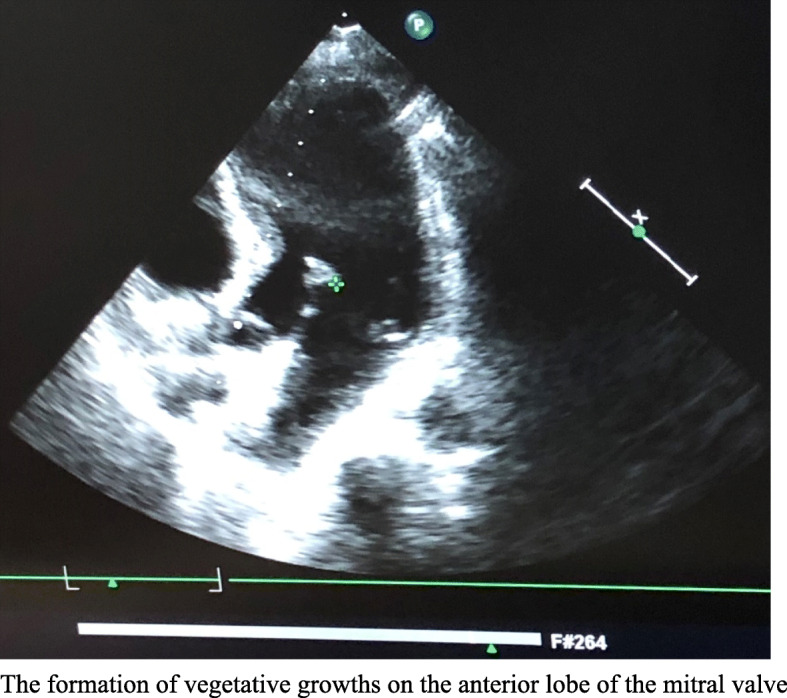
Transoesophageal echocardiography: The formation of vegetative growths on the anterior lobe of the mitral valve
